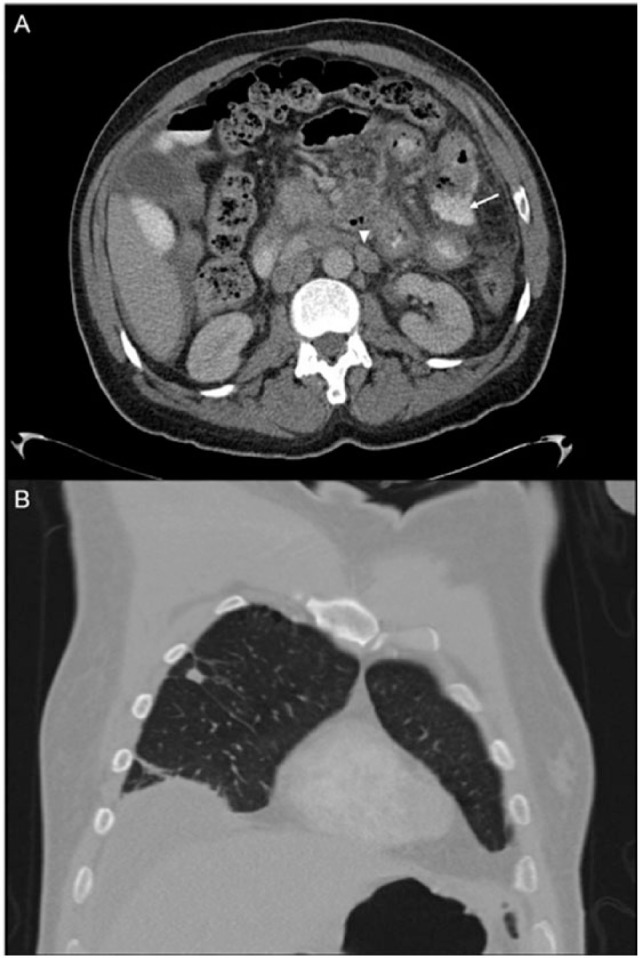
Computed tomography scans showing (A) extravasated oral contrast (arrow) and an enlarged periaortic lymph node (arrow head) and (B) a 1.1 cm noncalcified, nonspiculated pulmonary nodule of the right upper lobe.
